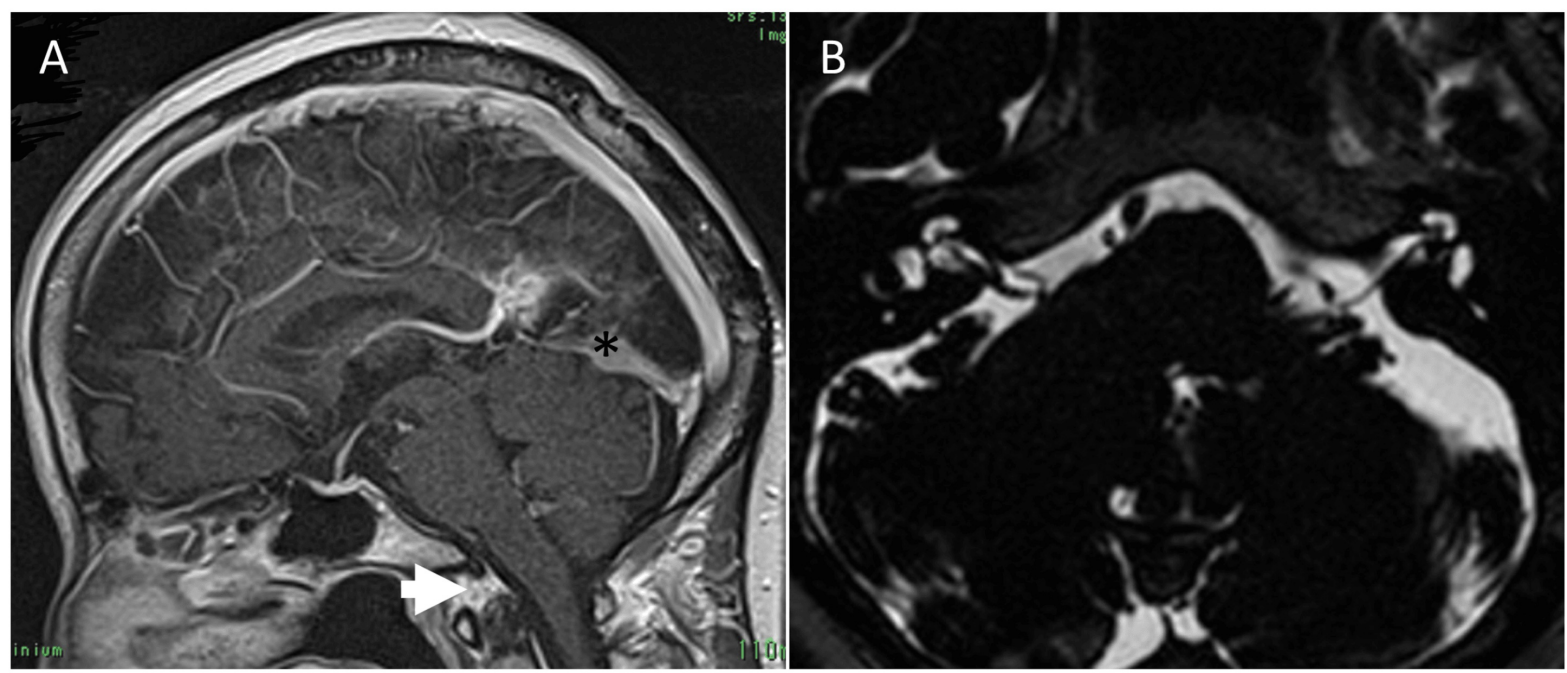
Postoperative magnetic resonance (MR) images Postoperative MR images were taken seven years after surgery. (A) is a sagittal contrast-enhanced T1-weighted image, and (B) is an axial image acquired using constructive interference in steady-state (CISS). The residual tumor (asterisk) did not increase in size after seven years postoperatively. The improved overcrowding at the foramen magnum (arrow) has also remained without recurrence. In the CISS image, the narrowing of the cerebellopontine angle cistern has not recurred.
